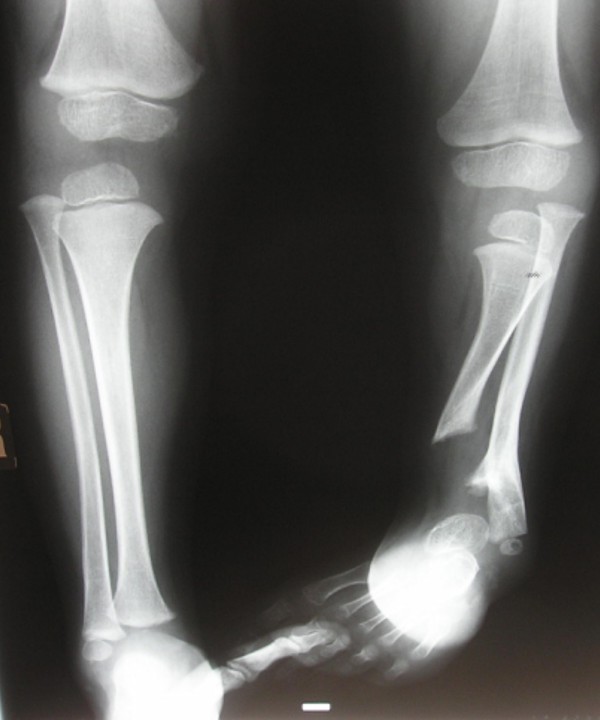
Anteroposterior radiograph of the lower limbs showed profound unilateral tibiofibular hypoplasia with subsequent development of ball and socket ankle joint secondary to fibular-tarsal dysplasia. Dysplasia of the tarsal and the metatarsals and hypoplasia of the terminal phalanges were evident. There was relative left tibial hypoplasia.
